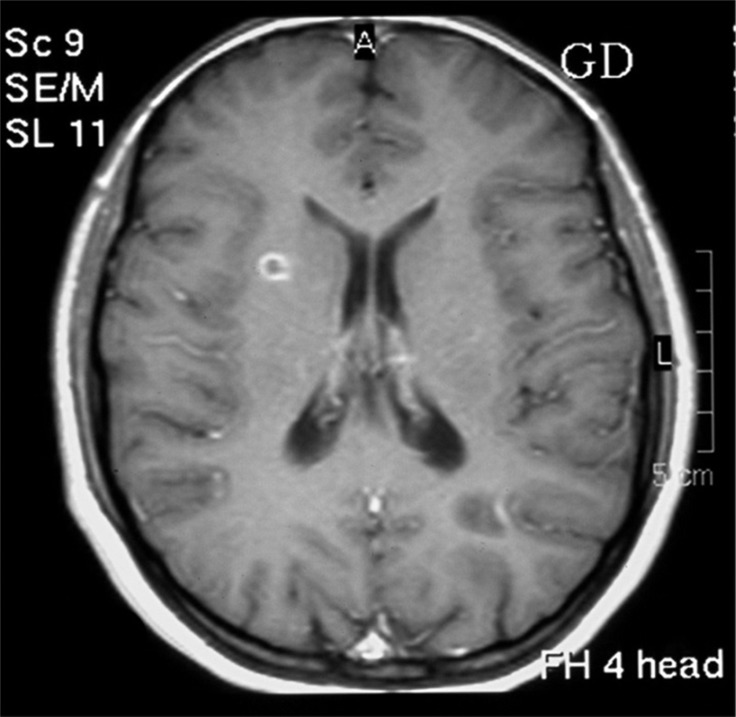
Brain magnetic resonance imaging, T_1_-weighted image showing ring-like enhancement of the active plaque
